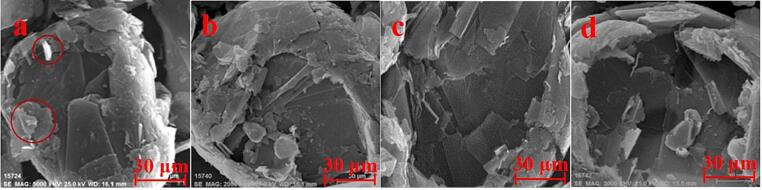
SEM images of graphite flotation concentrate pretreated by different methods (a: graphite ore; b: horn-type ultrasound, intensity of 100%, 1 min; c: bath-type ultrasound, intensity of 100 %, 4 min; d: combination of ultrasounds with horn-type ultrasound intensity of 100% and bath-type ultrasound intensity of 60%, 1 min).
